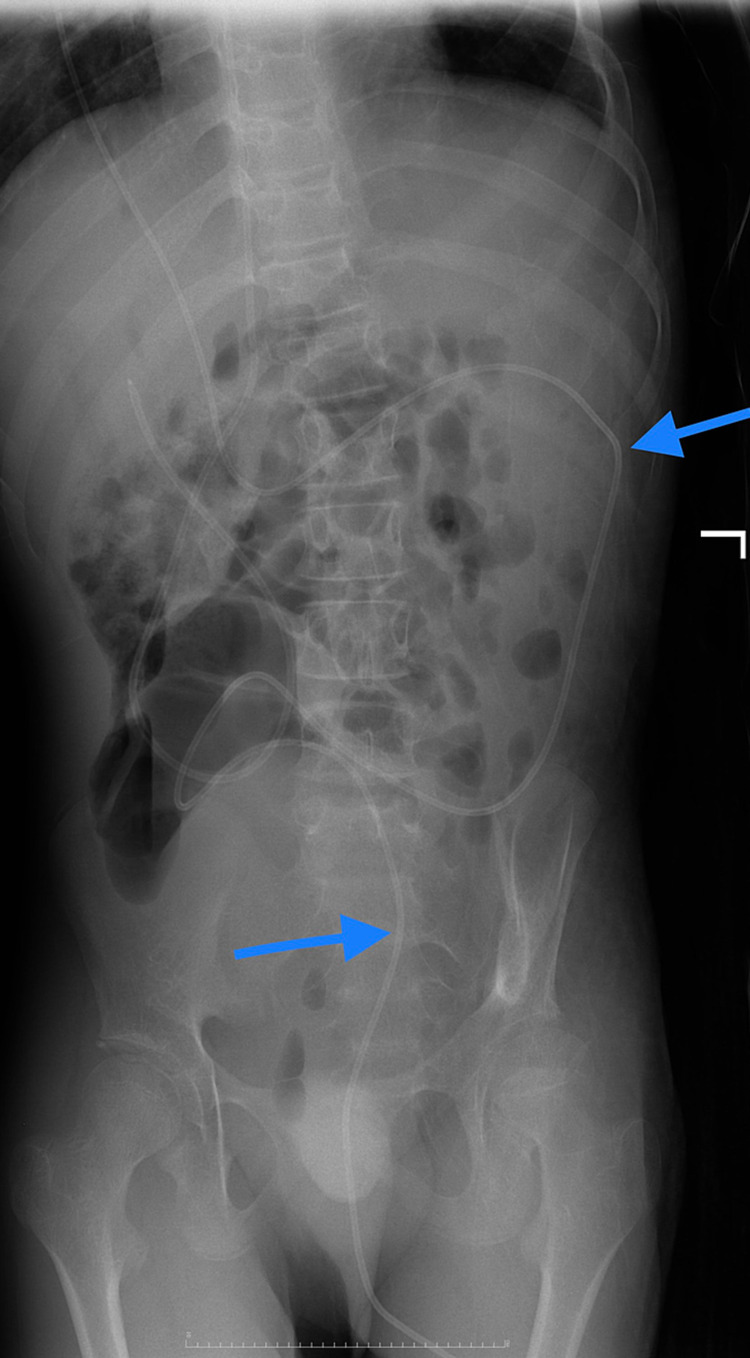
X-ray abdomen AP view - the blue arrows refer to the course of the catheter from the transverse colon down to the sigmoid colon, rectum, and anal canal.
